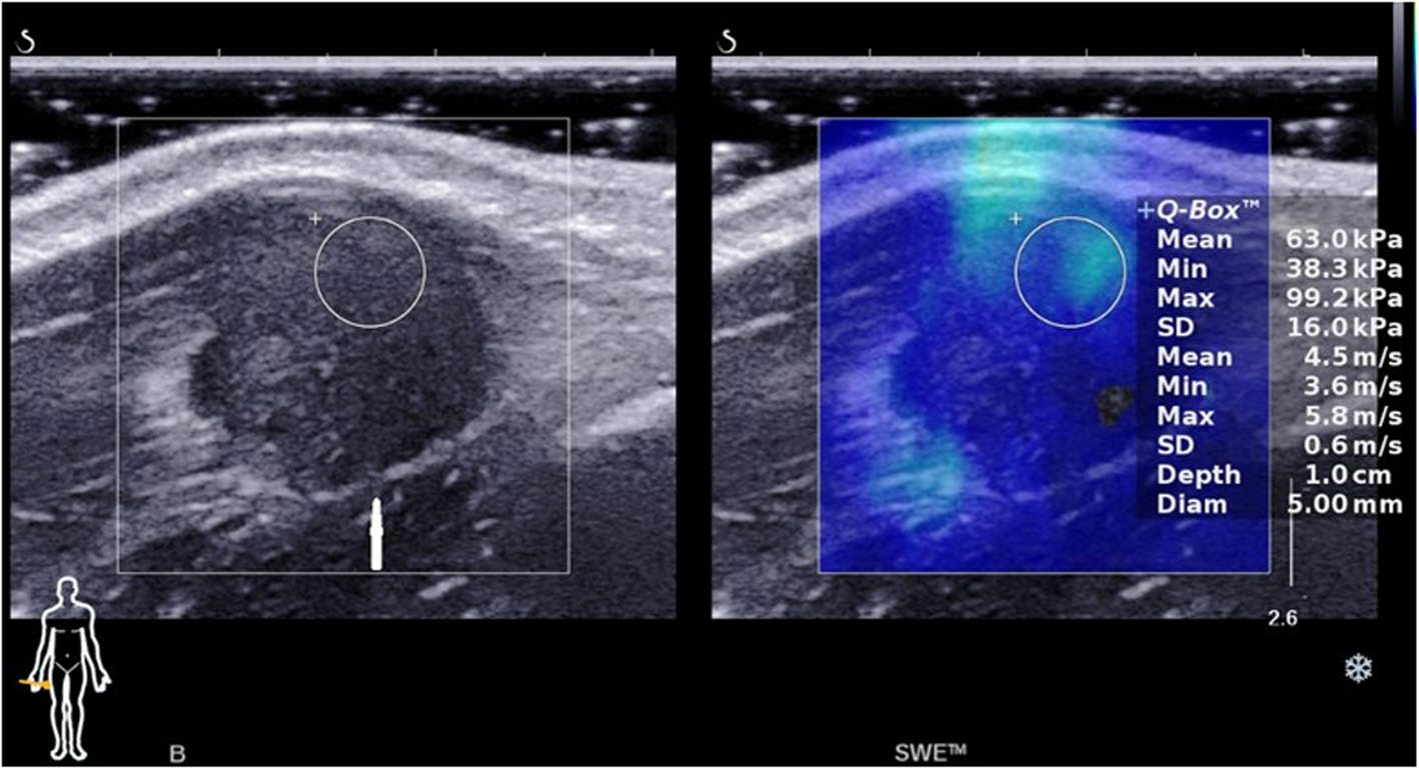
Gray-scale ultrasonography (left) shows a well-circumscribed homogeneous hypoechoic mass (arrows). Shear wave elastography (right) shows that the mean value of Young’s modulus is 63 kPa
